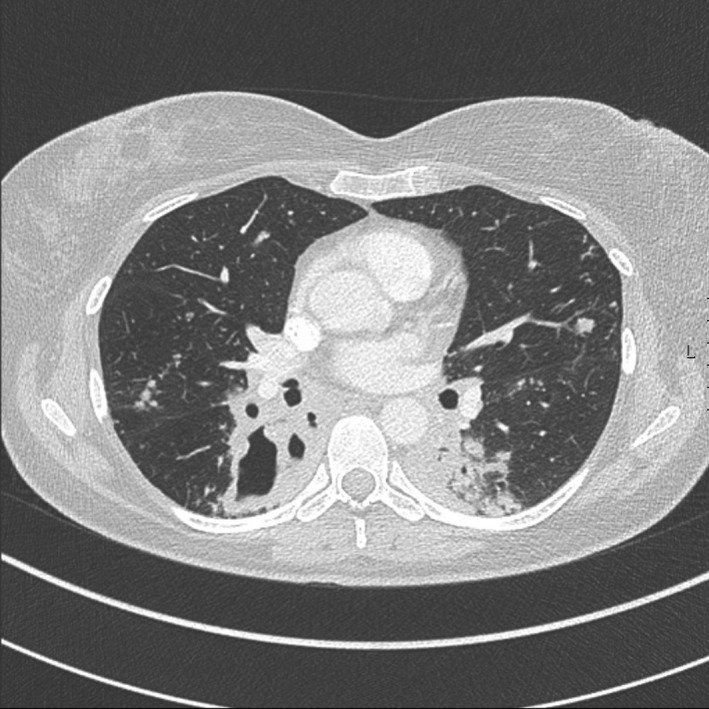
Thorax CT, lung window. Pulmonary cavitation in right lower lobe is present, demonstrating parenchymal destruction.
